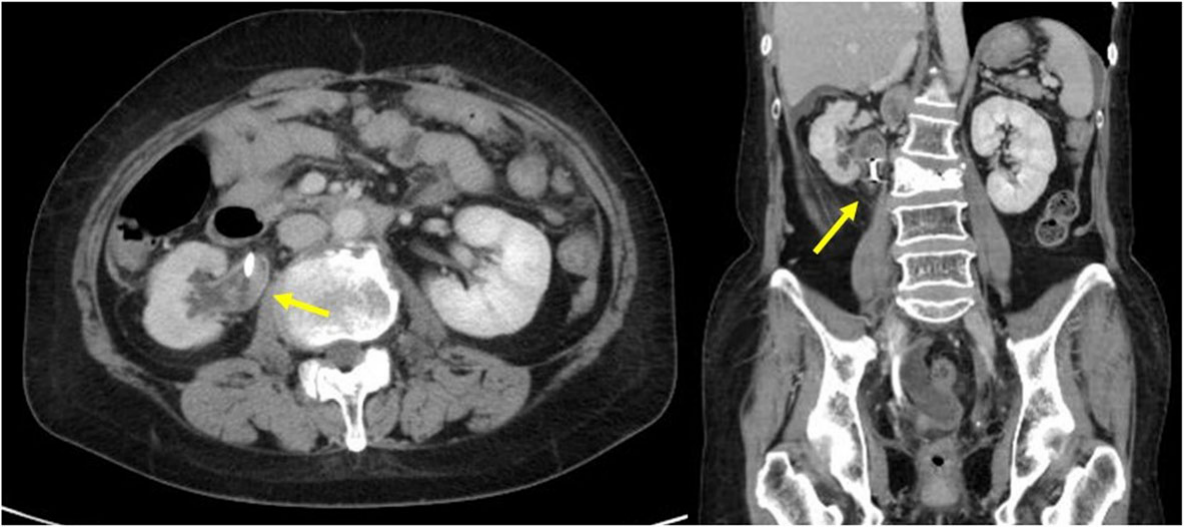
Contrast enhanced computed tomography showed a hematoma at the right ureteropelvic junction (arrows) but did not reveal a bleeding source
